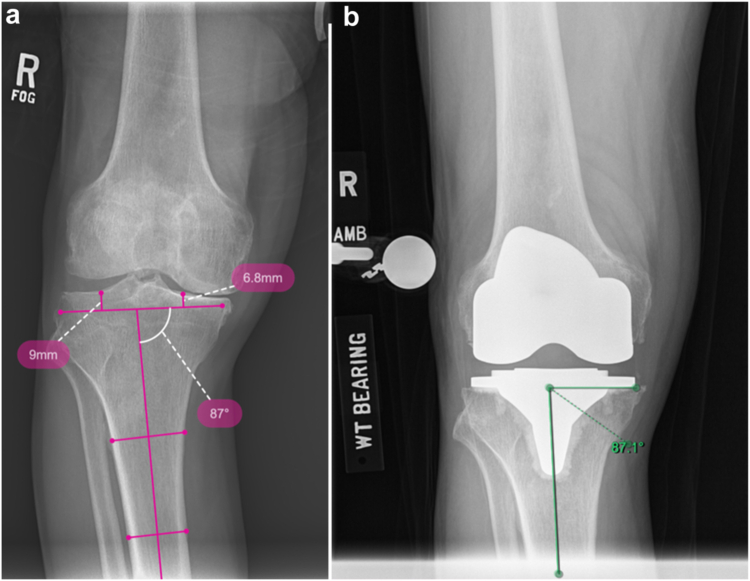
An alternative method involves preoperative templating of the MPTA and direct execution of this measured angle intraoperatively. (a) In this case example, the preoperative MPTA was determined to be 3° of varus. (b) Postoperative radiographs confirm restoration of the 3° tibial joint line resection angle.
